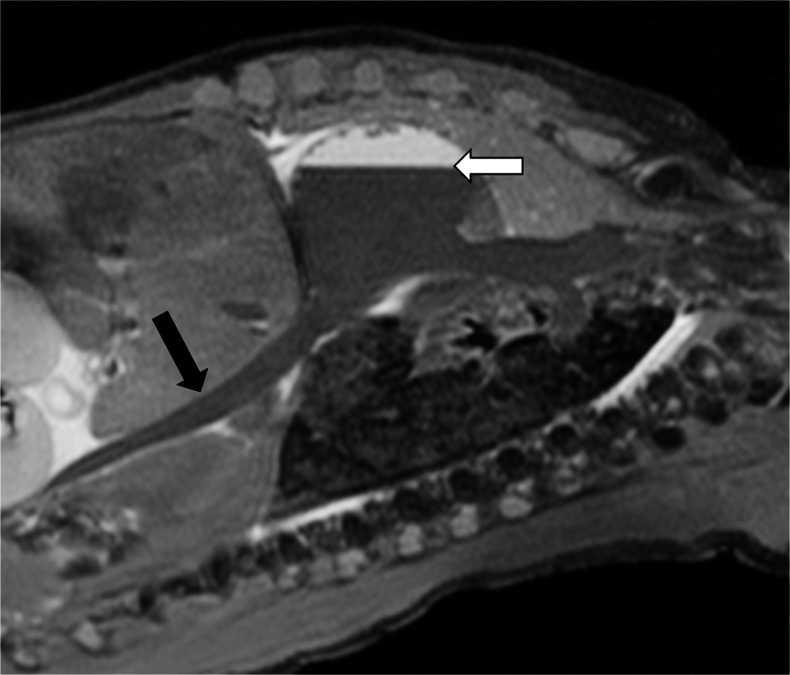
Blood layering. Sagittal T2-weighted postmortem magnetic resonance imaging in a term stillbirth. There is gravity = dependent sedimentation or layering of blood in the heart, shown as a fluid-fluid level (*white arrow*), which is frequently seen. Note gradual tapering and collapse of the inferior vena cava due to abdominal organ compression (*black arrow*), which is not pathological
